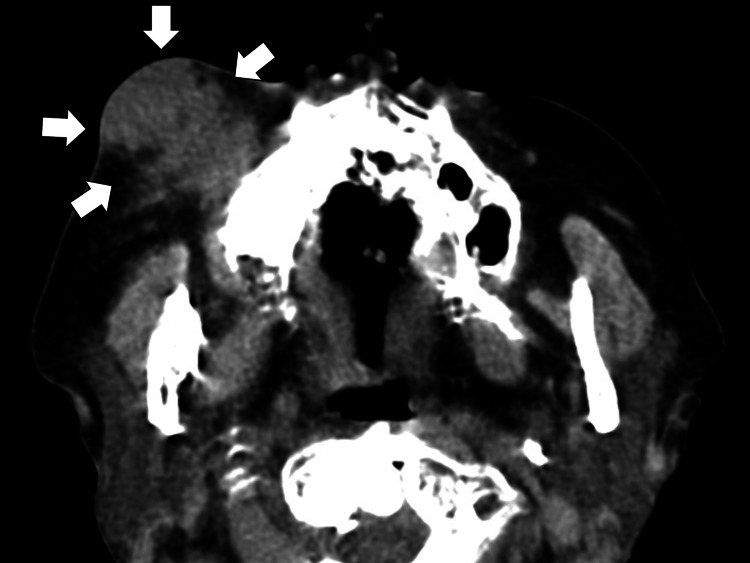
The facial CT revealing a suspected abscess anterior to the right maxillary sinus (white arrows)
